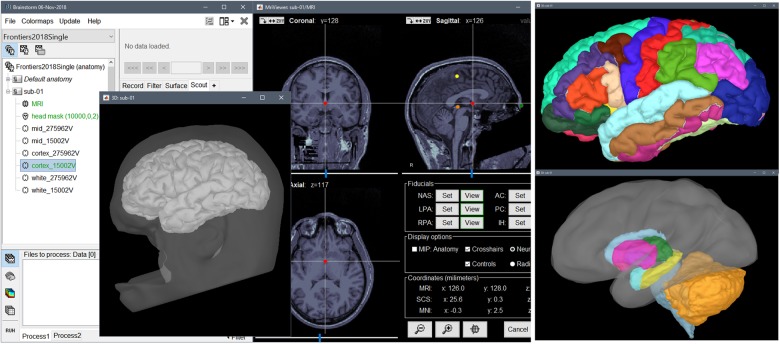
Brainstorm screenshot after importing anatomical MRI data and FreeSurfer derivatives. From (left) to (right): Brainstorm database explorer, 3-D rendering of head and pial surface meshes (the scalp surface and MRI volume were de-faced for anonymization purposes), orthogonal views of T1 MRI volume and anatomical fiducials, FreeSurfer/Mindboggle cortical parcellation, FreeSurfer/ASEG volume atlas of subcortical and cerebellar structures.
